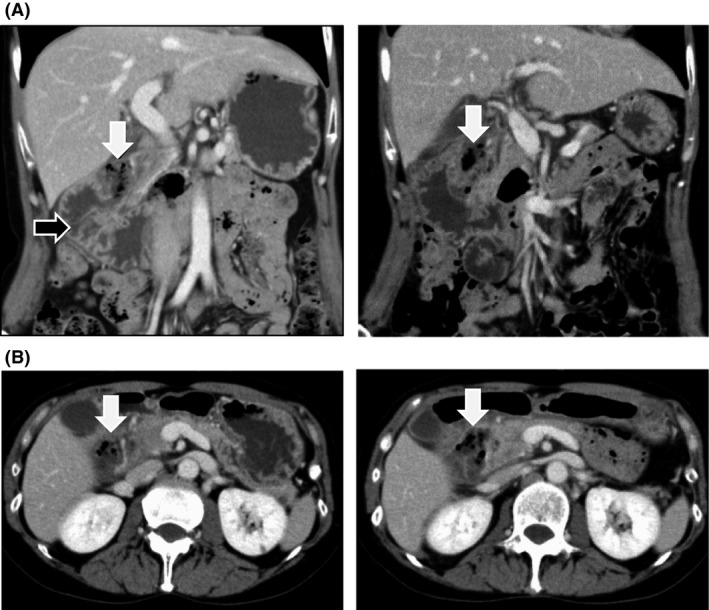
Coronal (A) and axial (B) section images by CT scans on the day of admission (the 1st day, left panels) and the 3rd day (right panels) showed an increase of retroperitoneal abscess (white arrowheads) apparently connected to juxta‐papillary diverticulum. The dilation and wall thickening of the descending part of duodenum is also indicated (black arrowhead).
